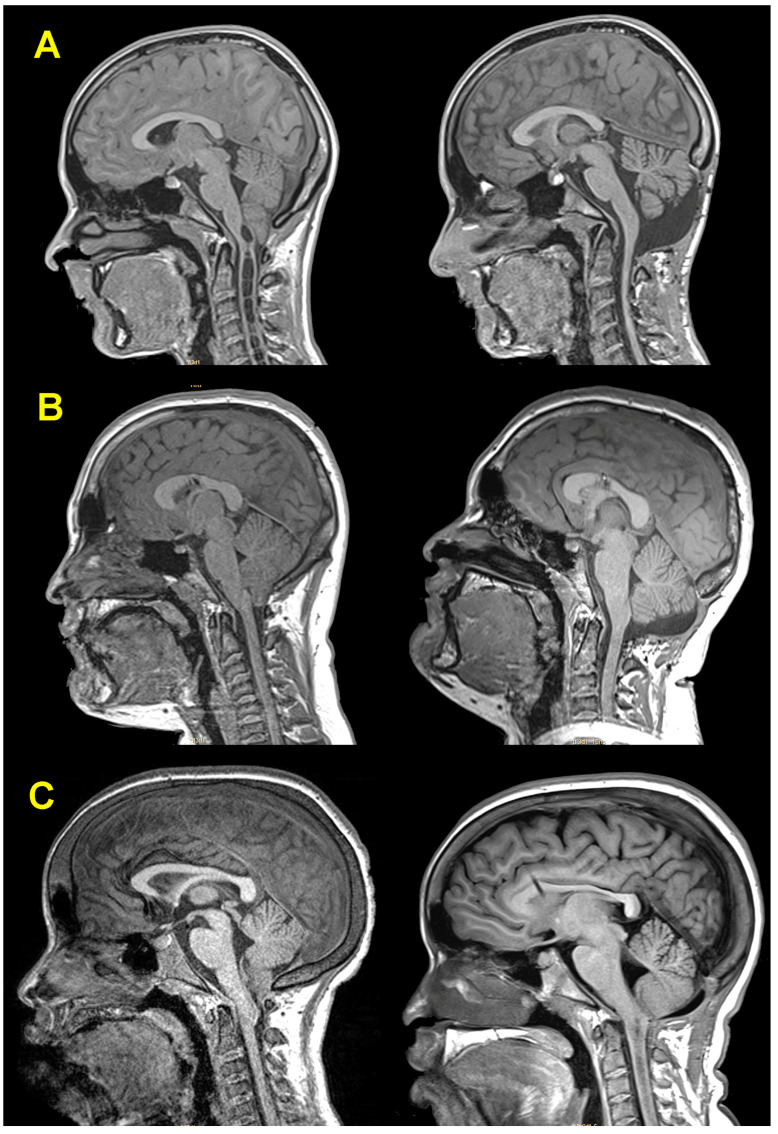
Surgical outcomes. The figure shows three representative patients of those considered as having “very good” (A), “good” (B), and “bad” (C) surgical results. In patient (A), there was complete relief of presurgical symptoms and disappearance of the syrinx cavity, with an important improvement of the scoliosis (surgical outcome = very good). In patient (B), despite the correct formation of a neocisterna magna after posterior fossa reconstruction (PFR), we considered that the clinical result had not been optimal because the child did not improve from the pre-surgical papilledema. The presence of persistent papilledema, without headaches, despite the ventriculoperitoneal CSF shunt being revised on several occasions, motivated the performance of PFR to be able to implant a lumbo-peritoneal CSF shunt. In this case, the surgical result, which combines the clinical changes with neuroimaging changes, was classified as “good”. Patient C is one example of the four patients with a bad surgical outcome: PFR was carried out in a child with sickle cell anemia and headaches precipitated by Valsalva maneuvers; the child had acute hydrocephalus three weeks after the PFR and needed a shunt. The image on the right shows the control MRI showing local arachnoid scarring and the presence of an asymptomatic syringomyelic cavity that was not present before the PFR.
